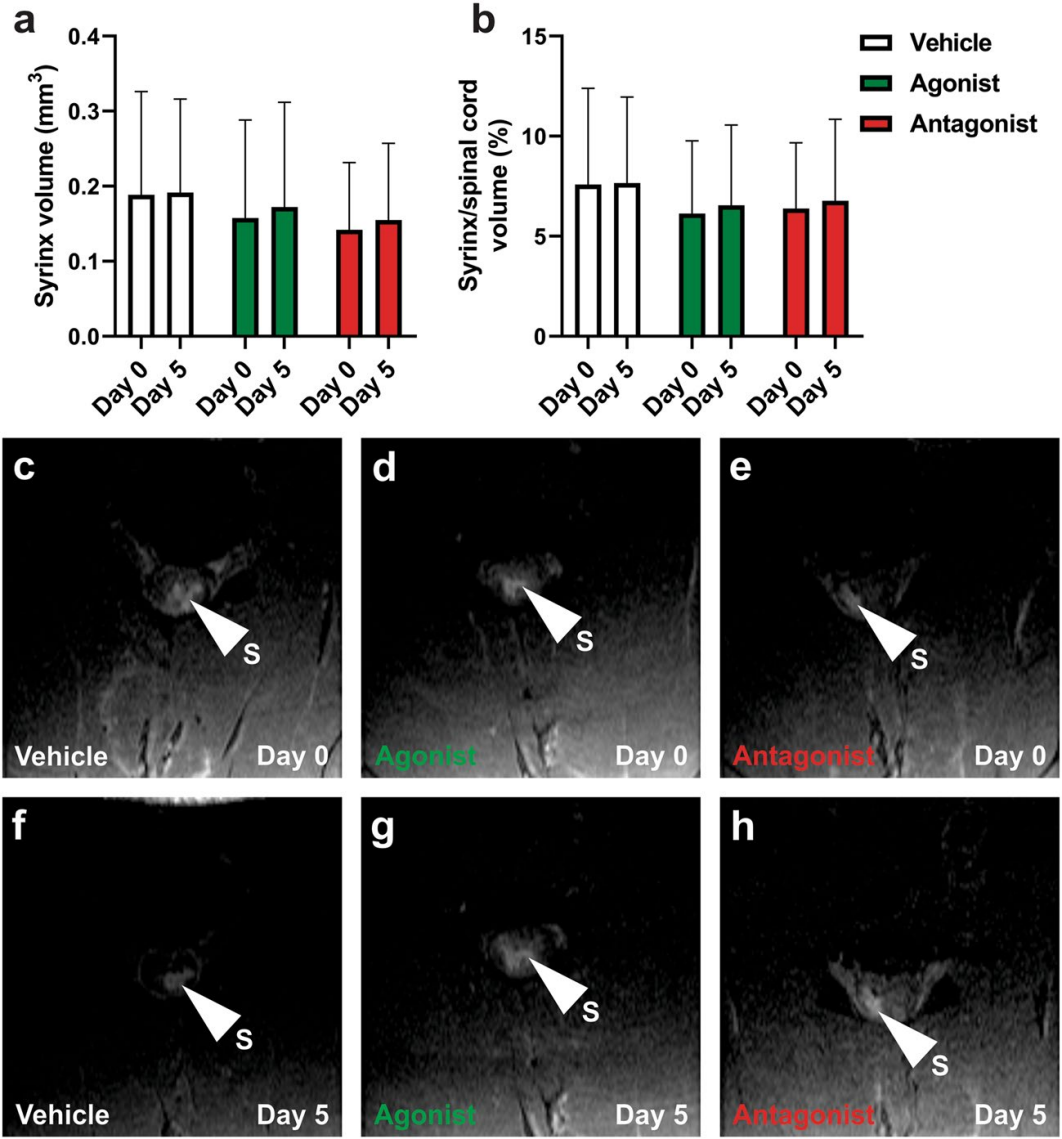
Syrinx volume quantification on MRI before and after modulation of AQP4 activity. (a) Absolute syrinx volume and (b) syrinx volume as a percentage of the respective whole spinal cord volume were taken before (day 0) and after (day 5) modulation with AqF026 (agonist; n = 9), AqB050, (antagonist; n = 10) and DMSO in saline (vehicle; n = 10). (c–h) Representative MRIs in the axial plane for vehicle (c, f), agonist (d, g) and antagonist (e, h) at day 0 (c–e) and day 5 (f–h). The white arrowhead indicates the syrinx (S), which appears as a hyperintense region on T2-weighted MRI. Results in (a) are mean ± SD (two-way ANOVA, *p* = 0.738) and (b) are mean percentage ± SD (two-way ANOVA, *p* = 0.790).
